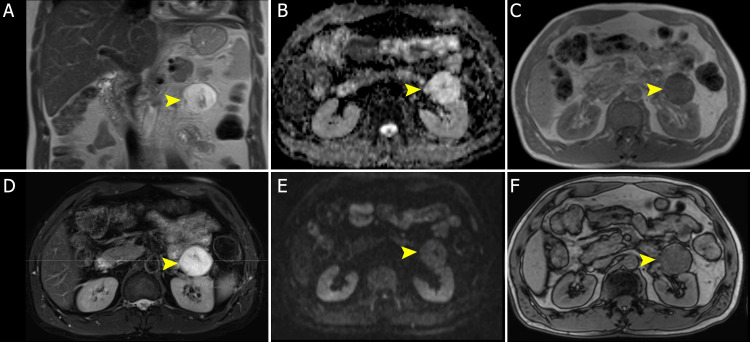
Abdominal MRI part 1 Coronal T2-weighted sequence (A) and axial fat-suppressed T2-weighted sequence (D) show a very high signal-intensity mass, similar to the one from cerebrospinal fluid. The mass has no diffusion restriction in the ADC map (B) and the b800 DWI sequence (E). The mass has no signal drop in the dual-echo in- (C) and opposed-phase T1-weighted imaging (F). ADC: Apparent diffusion coefficient, DWI: Diffusion-weighted imaging
